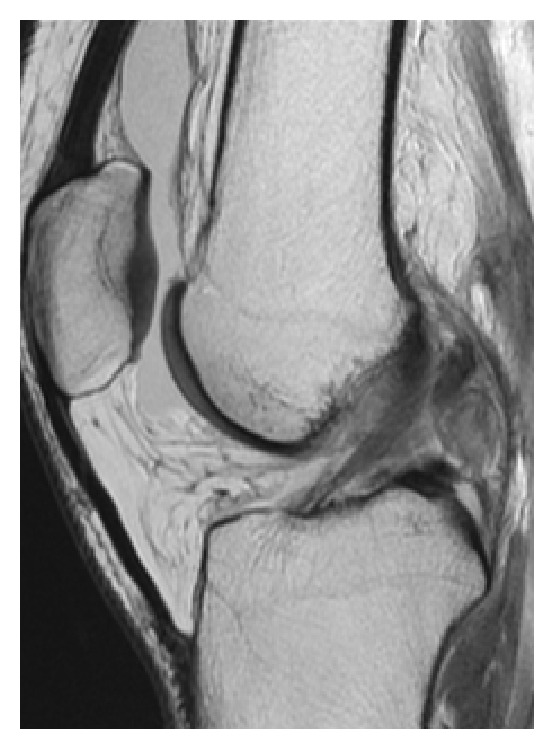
24-year-old female with localized PVNS at the posterior cruciate ligament (case #P5). Bursa suprapatellaris is clearly distended with fluid. Sagittal proton density-weighted MR image.
